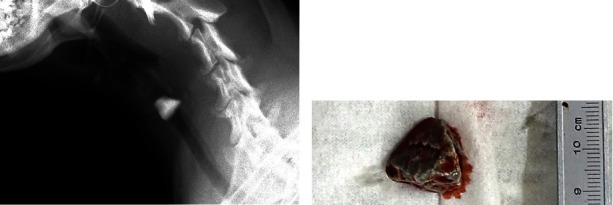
A large foreign body (angular stone) in the proximal trachea with near-complete lumen obstruction in case 1. Right lateral radiographs of the cervical area before surgery (a) and gross appearance of the removed stone (b).
